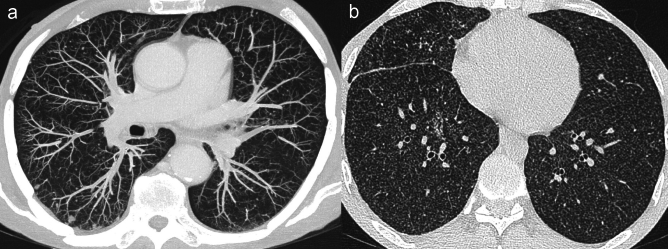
Miliary distribution of lung metastases versus miliary tuberculosis. a Miliary metastases in a 72 y.o. man with gastric carcinoid tumour endoscopically resected. Axial computed tomography (CT) maximum intensity projection (MIP) image shows multiple metastatic micronodules with a miliary distribution and variable size. Note the feeding vessel sign in the two larger nodules located in the right lower lobe. b Miliary tuberculosis in a 33 y.o. man. Axial CT scan depicts multiple micronodules widely disseminated throughout the lungs
